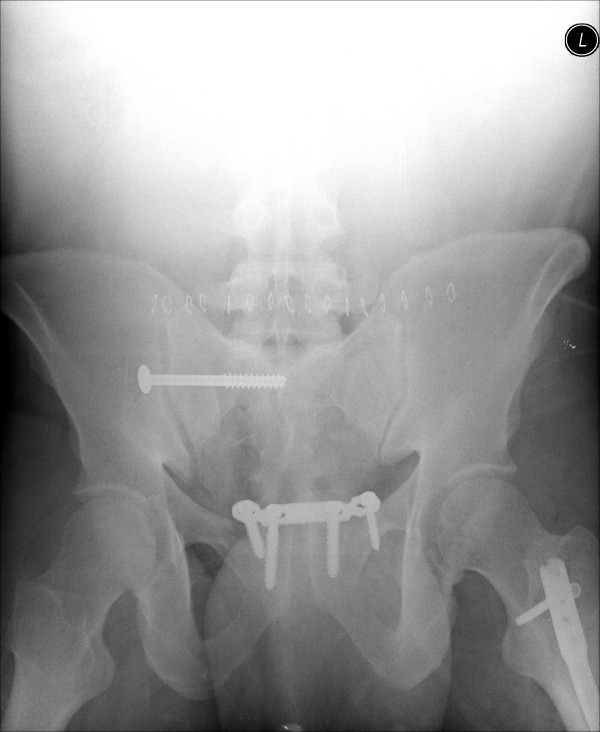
Immediate postoperative outlet radiograph showing satisfactory symphyseal and sacroiliac joint reduction and fixation.
